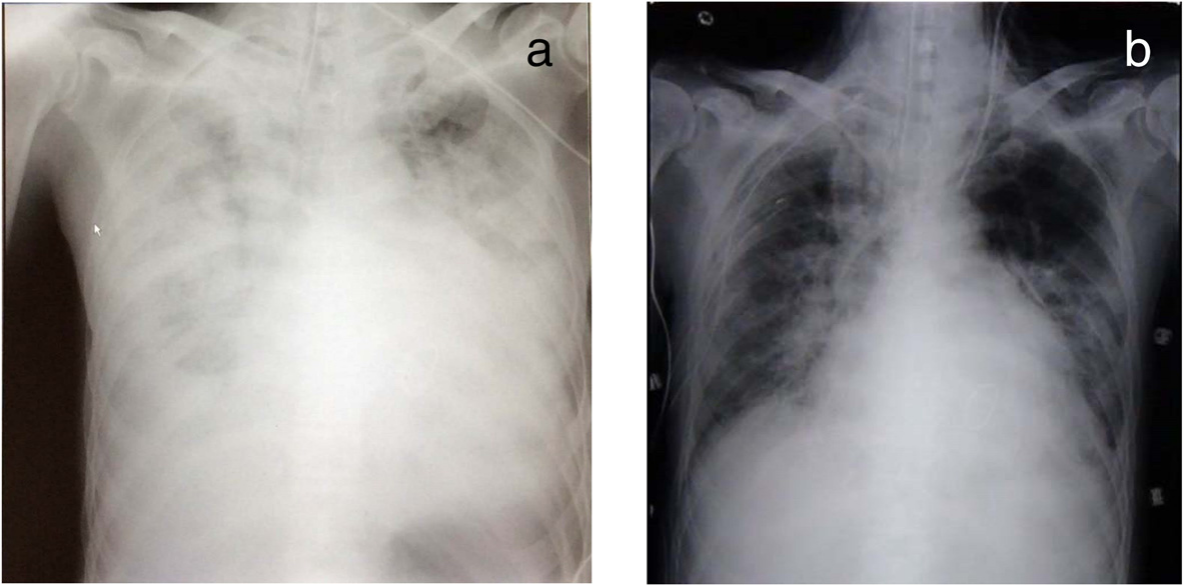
Chest radiographs at ICU admission (a) and at weaning from ECMO (b)
